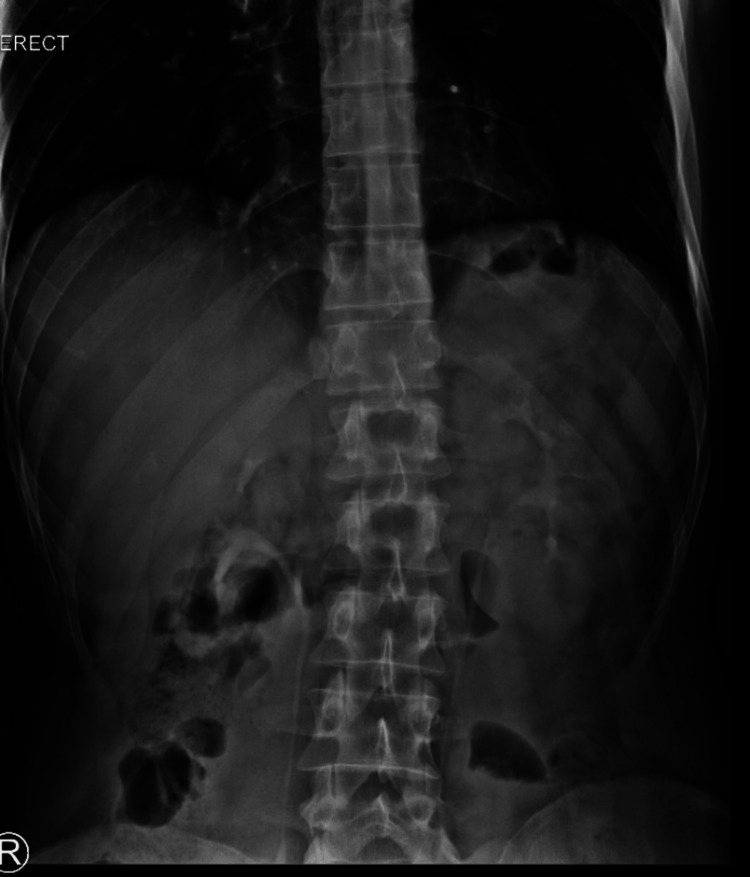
A plain film of the abdomen showing usual gas pattern.
